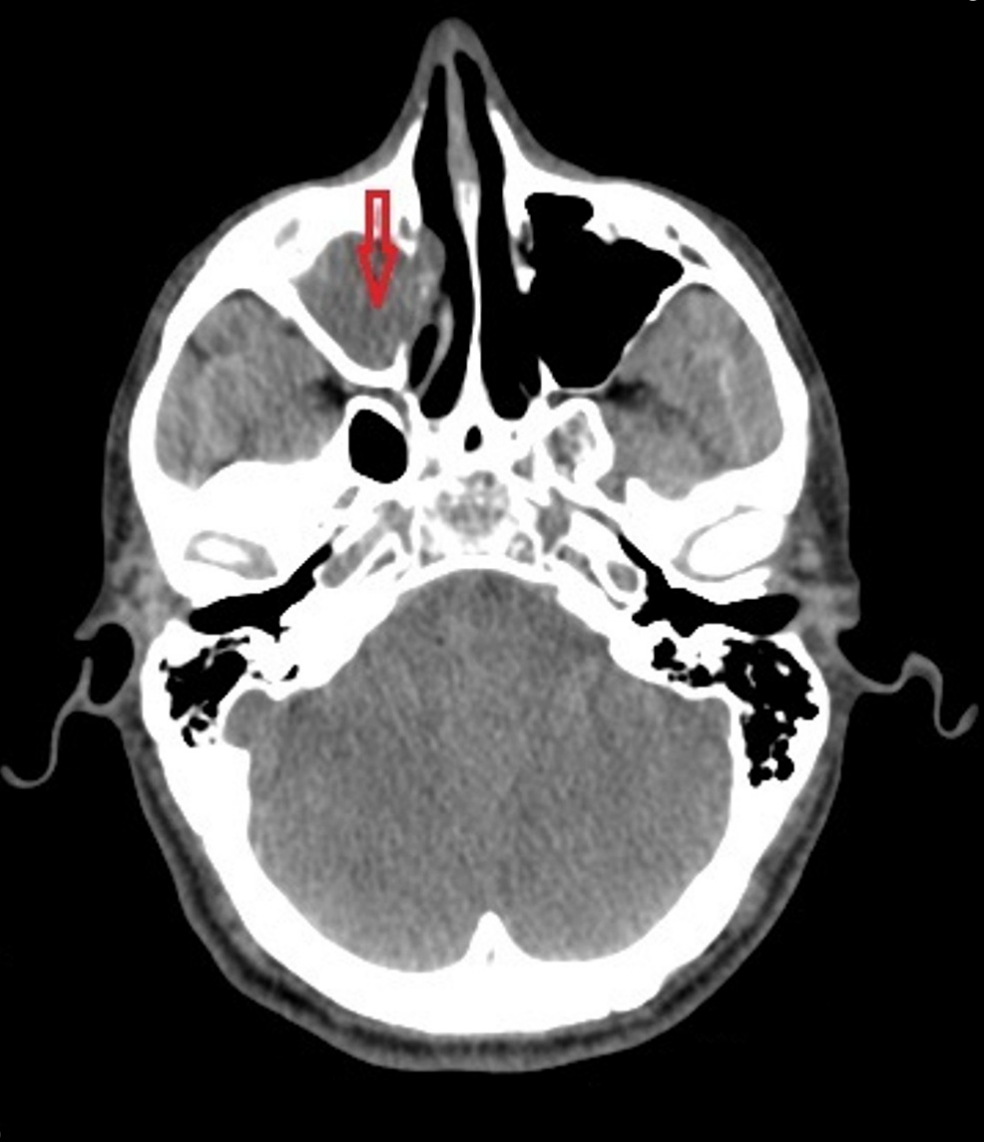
CT demonstrating opacified right maxillary sinus.
